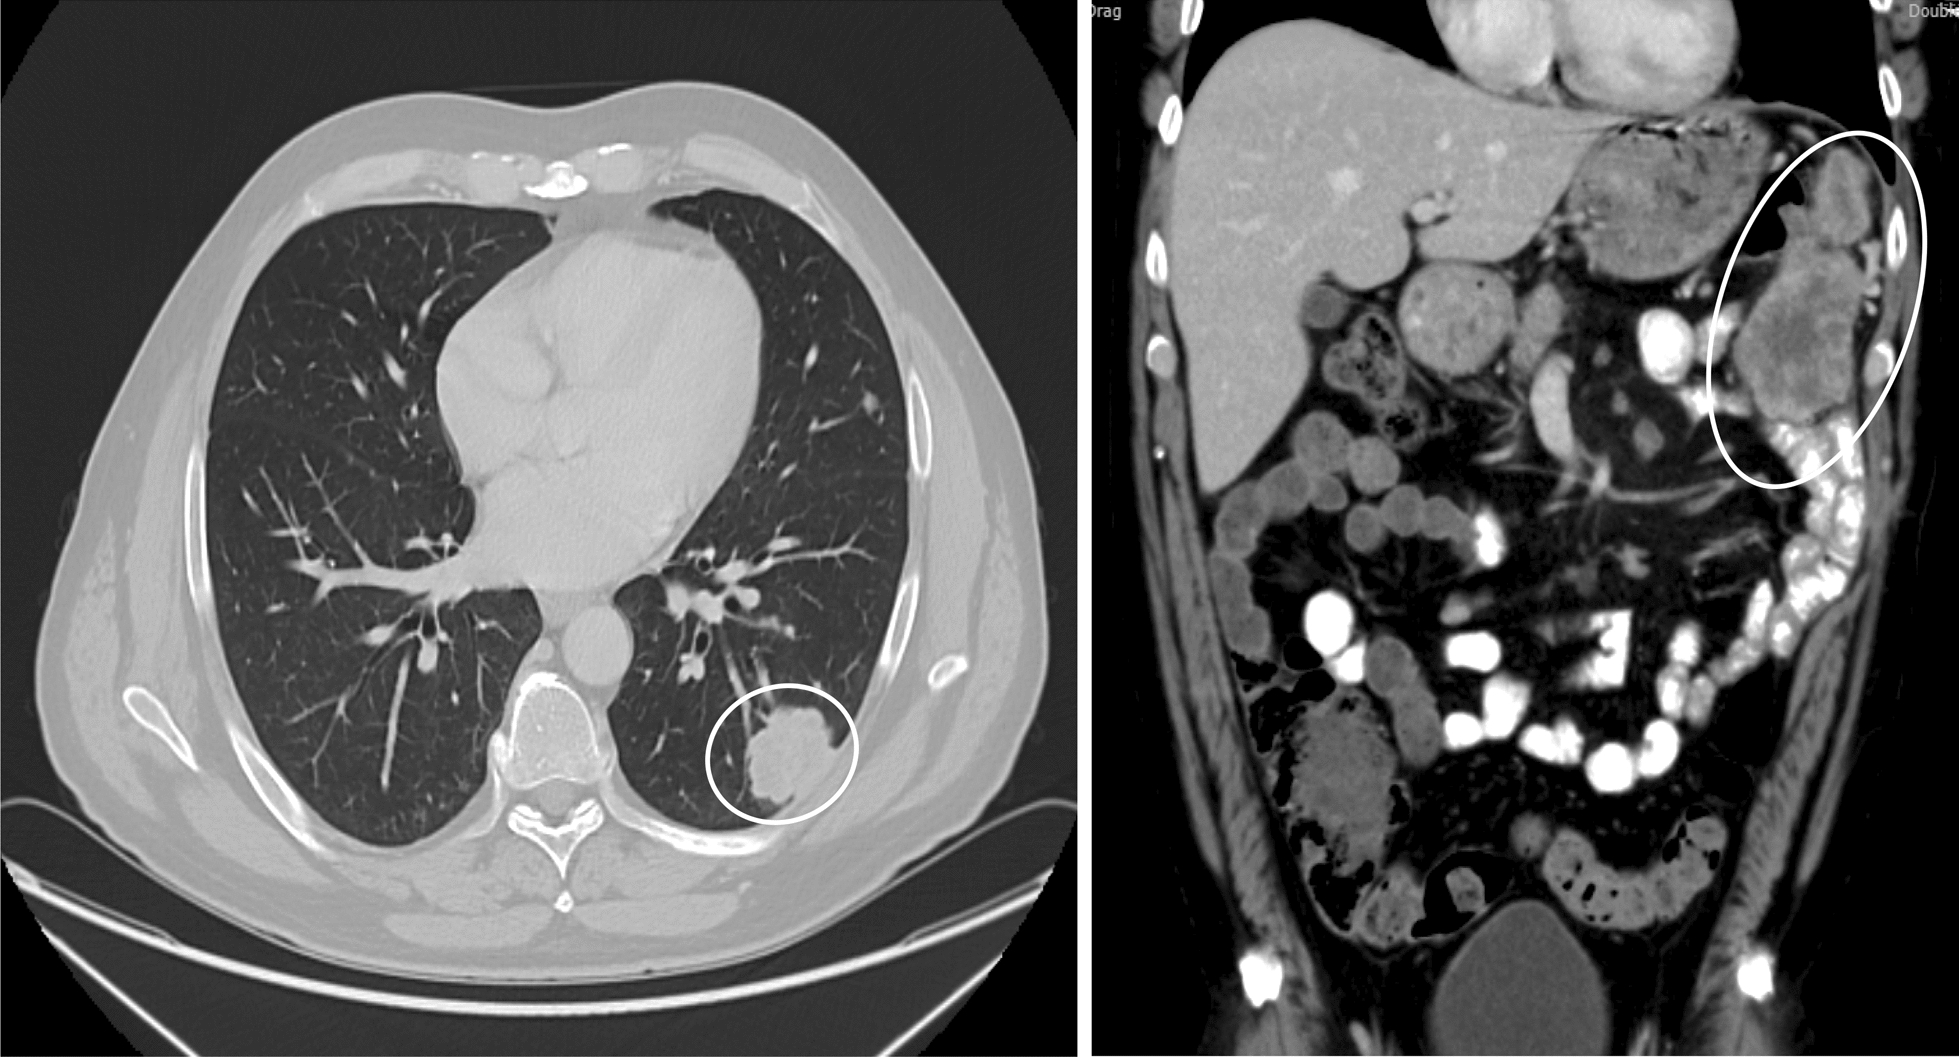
Baseline contrast-enhanced computed tomography images obtained in 2018 prior to nivolumab initiation. A Axial chest computed tomography demonstrating a subpleural intrapulmonary metastatic lesion with a lobulated contour in the left lung (highlighted by a circle). B Axial abdominal computed tomography showing a 3.0 cm × 2.4 cm metastatic mass in the lower segment of the spleen, with additional metastatic lesions detected around the spleen, between the intestinal loops, and beneath the abdominal wall (highlighted by ovals)
